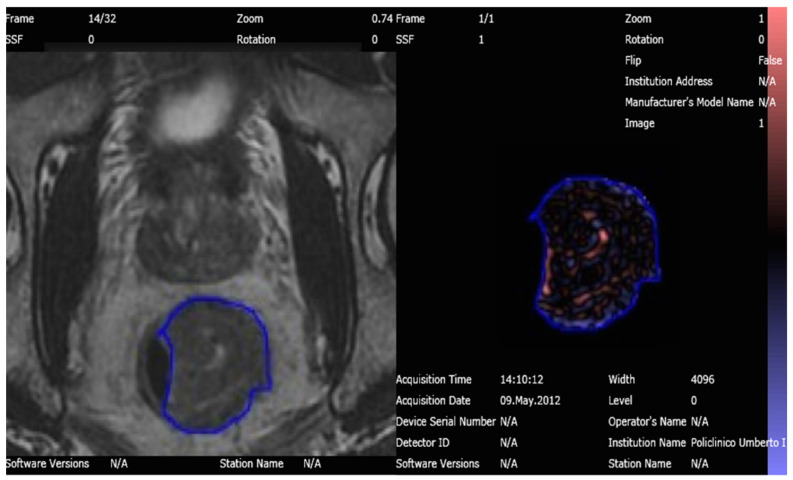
T2 Weighted-Image on 3T MRI of the rectal tumor before nChRT segmented and analyzed with Texture Analysis software.
